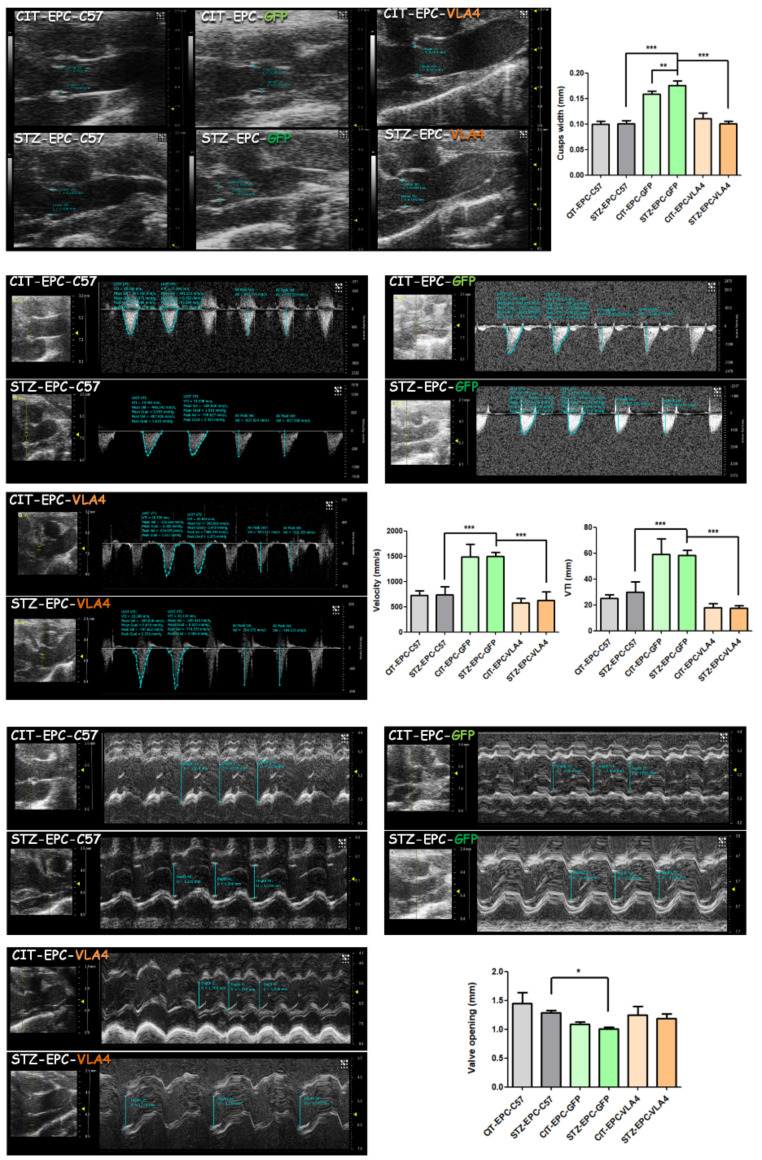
Morphologic and functional echocardiographic measurements. Shown are representative images of B-mode measurements of leaflets width and subsequential statistical analysis (Upper panel); pulsed-wave Doppler mode (PW Doppler-mode) measurements of the left ventricle outflow tract (LVOT), velocity-time integral (VTI) and Velocity (Middle panel); and M-mode measurements of maximum systolic valve opening (Lower panel). ANOVA, Bonferroni post-test were applied: * *p* < 0.05,  *p* < 0.01, * *p* < 0.001.
